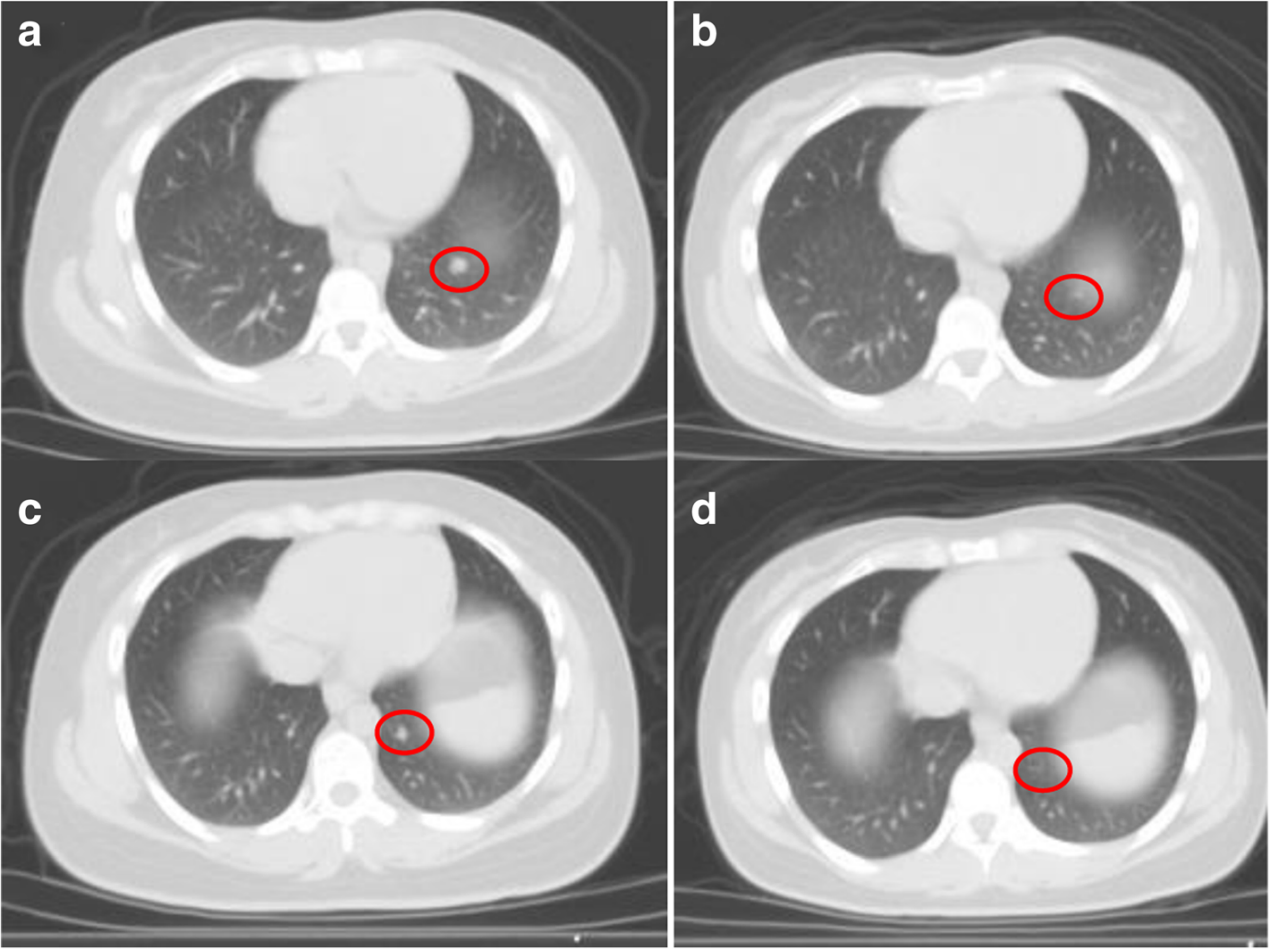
Axial CT demonstrating interval improvement in the metastatic lung lesions in patient 1 after 5 cycles of Nivolumab. a Left lower lobe nodule #1 prior to Nivolumab. b Left lower lobe nodule #1 after Nivolumab. c Left lower lobe nodule #2 prior to Nivolumab. d Left lower lobe nodule #2 after Nivolumab
